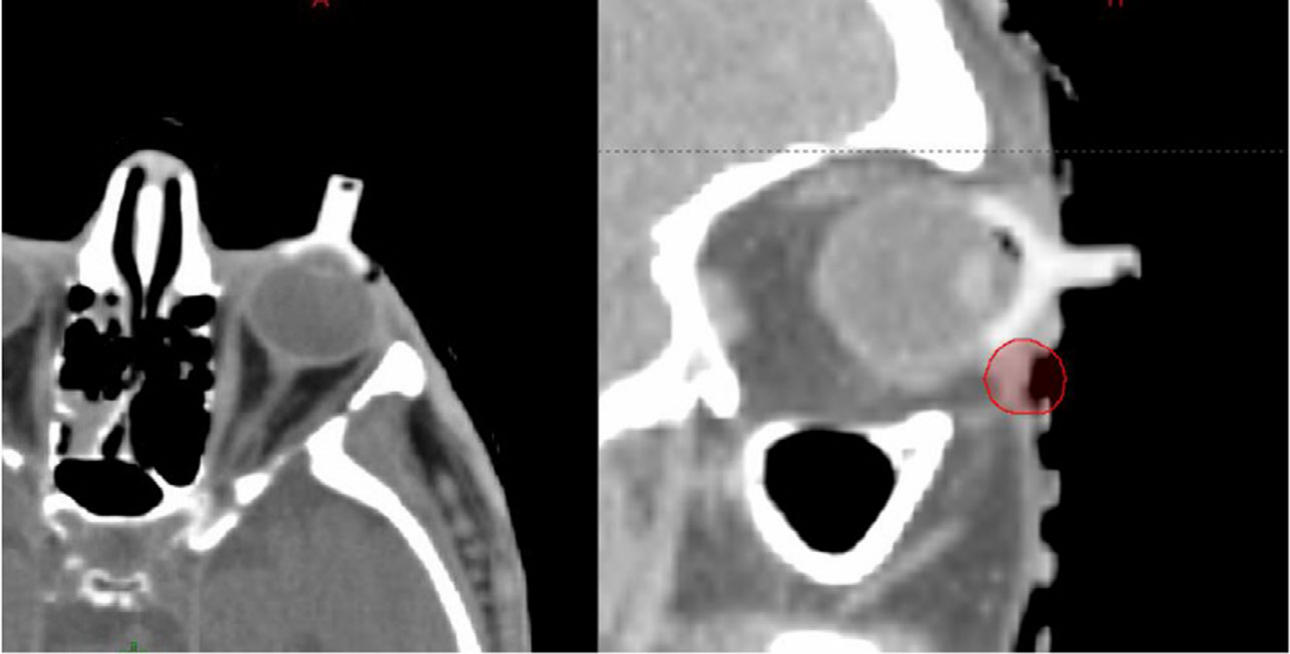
Dummy eye shield inserted for patients CT scan. Red circle is the clinical target volume.
